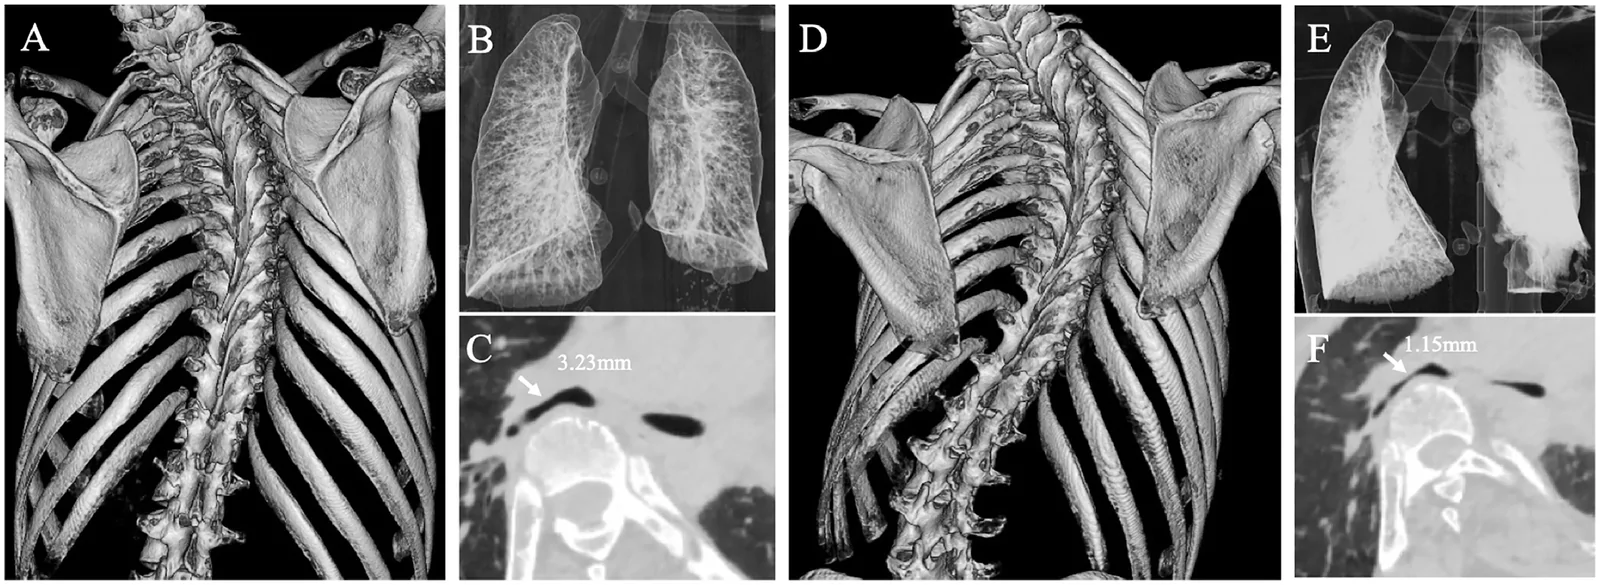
Preoperative multidetector computed tomography (MDCT) in supine and upright positions. (A) Three-dimensional reconstructed image of the thoracic cavity in the supine position obtained from MDCT. (B) Three-dimensional reconstructed image of the lung volume in the supine position obtained from MDCT. (C) Axial lung-window image of the right main bronchus in the supine position obtained from MDCT. (D) Three-dimensional reconstructed image of the thoracic cavity in the upright position obtained from MDCT. (E) Three-dimensional reconstructed image of the lung volume in the upright position obtained from MDCT. (F) Axial lung-window image of the right main bronchus in the upright position obtained from MDCT. Lung volumes measured on MDCT were 2541 mL in the supine (B) and 1794 mL in the upright position (D), indicating a 30% decrease upon standing. The right main bronchus was further compressed in the upright position (C: supine; F: upright), with the minimum luminal diameter decreasing from 3.23 to 1.15 mm (64.4% reduction).
